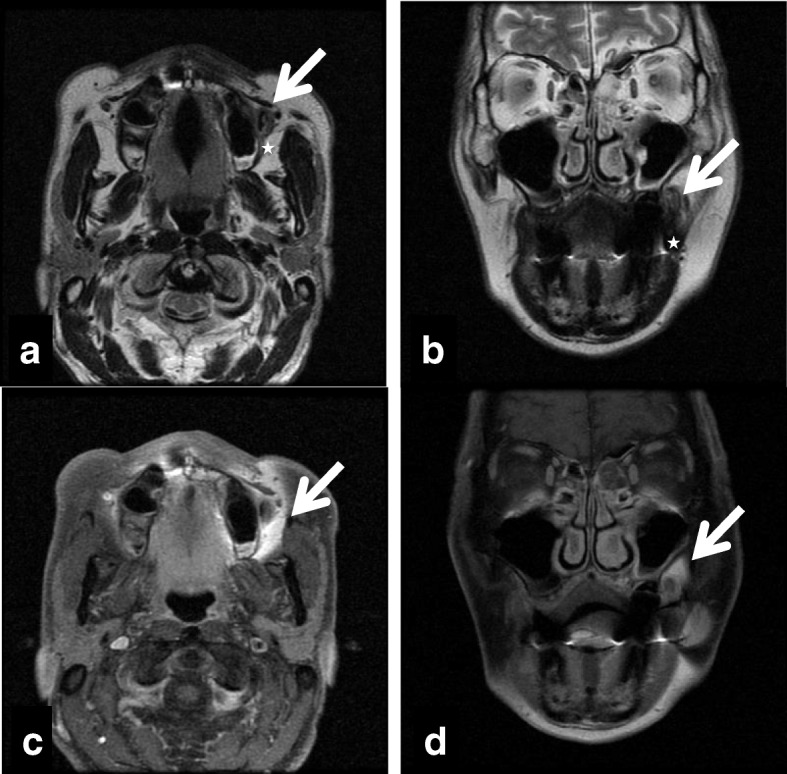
T2-weighted magnetic resonance images, a) axial view, b) coronal view, showing a pouch with an opening and a slightly thickened wall, and a tear in the buccinator muscle on the left side (white arrow). The five-point star indicates the buccinator muscle near the pouch. Gadolinium-enhanced T1-weighted magnetic resonance images, c) axial view, d) coronal view, revealing the wall demonstrating enhancement extending over the surrounding tissues antero-posteriorly in the buccal lesion and superiorly in the maxilla on the left side, which was considered to indicate an inflammatory reaction (white arrow)
